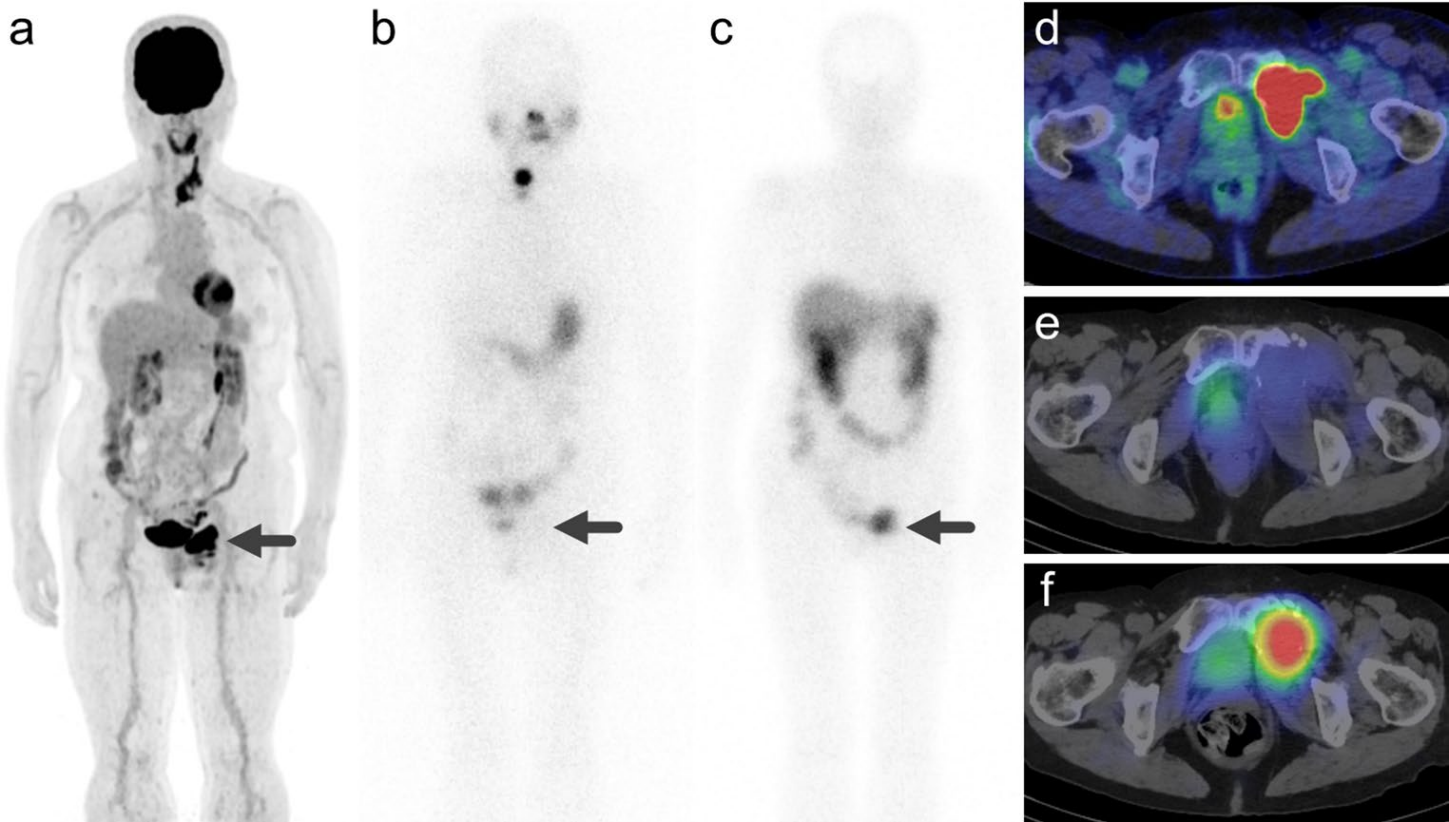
Representative case 2. A case of a woman in her 70s with papillary thyroid carcinoma and metastasis to the left pubic bone. (a) Maximum intensity projection of ^18^F-FDG PET showing increased uptake corresponding to the left pubic bone metastasis (Score 5/5, SUVmax = 12.2). (b) Post-treatment ^131^I scintigraphy performed at 3 days after ^131^I administration revealed no significant iodine uptake (Score 1/3, SUVmax = 0.04). (c) ^111^In-pentetreotide scintigraphy showing intense uptake, stronger than that in the liver, was observed (Score 3/4, SUVmax = 5.0). (d) Fused image of ^18^F-FDG PET and CT. (e) Fused image of ^131^I SPECT and CT. (f) Fused image of ^111^In-pentetreotide SPECT and CT
